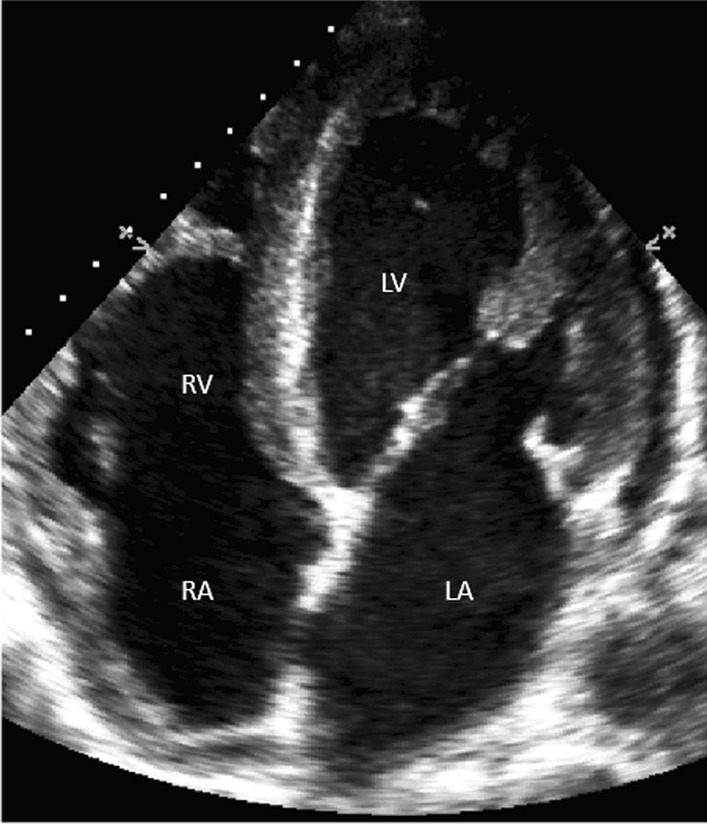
Heart ultrasounography. Apical four chamber view. *RA* right atrium, *RV* right ventricle, *LA* left atrium, *LV* left ventricle
